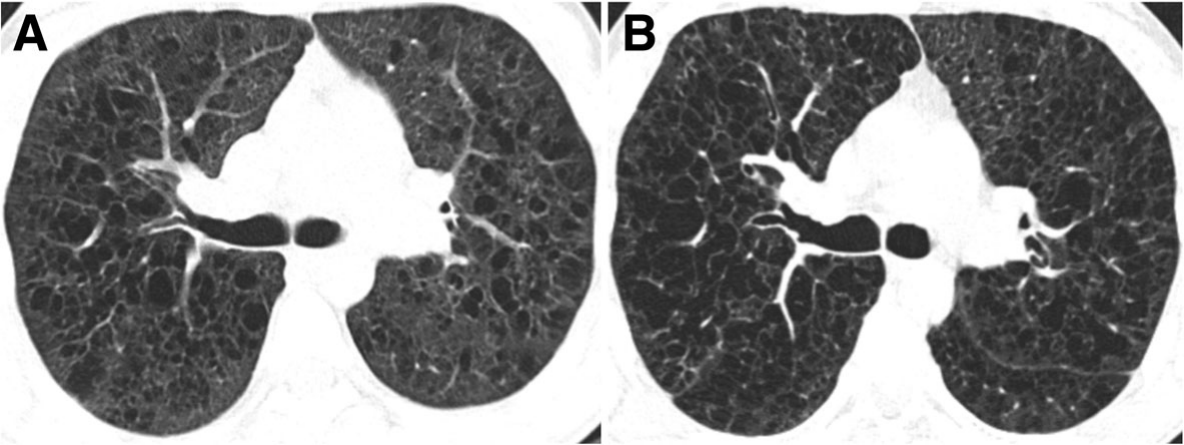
PLCH. Progression of the imaging findings over time (10-year interval), despite smoking cessation, in a 30-year-old man. a Axial CT image showing diffuse bizarre cysts with walls of varying thickness. b Axial CT image at 10-year interval showing progression of the number and size of the cysts in both lungs
